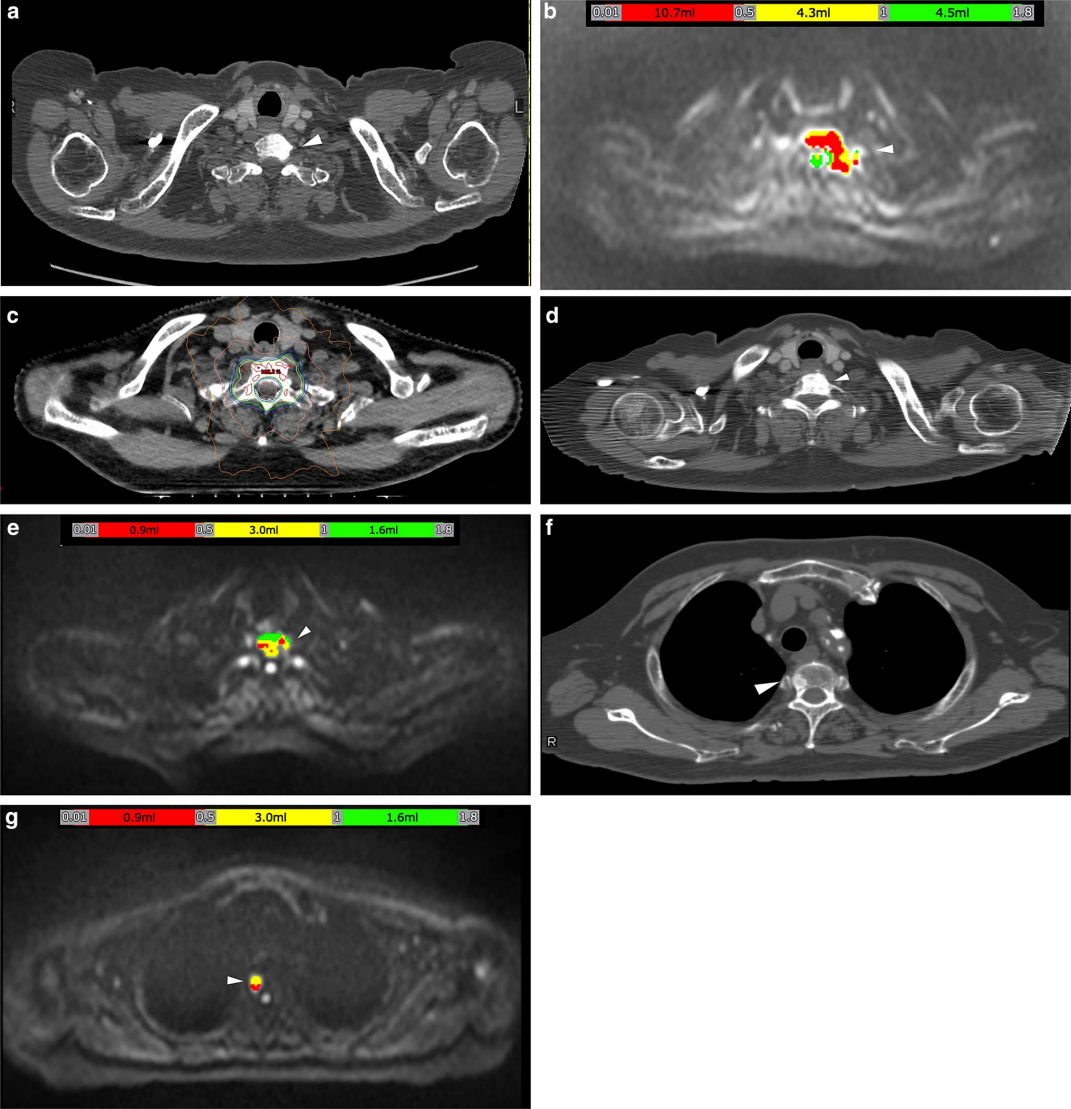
A 70-year-old man with CRPC. Six years ago, local radiotherapy was performed for prostate cancer (T2c N0M0, PSA 32.1 ng/mL, GS 4 + 5). Four years later, the patient’s condition transitioned to CRPC. a On the original axial CT at the level of C7, osteosclerotic change was seen in the left part of C7 body (white arrowhead). At that time, the patient’s PSA level was 4.413 ng/mL. b On the BD score–composed axial image at the level of C7, a colored area was seen (white arrowhead). tDV was 19.5 mL, and the mean ADC value was 0.74 (× 10^–3^ mm^2^/s). c The C7 lesion was regarded a metastatic lesion, intensity-modulated radiation therapy was performed (35 Gy/5fr RT.) with reference to the axial DW image. d–g Approximately 10 months after the image shown in a–c, PSA increased to 6.235 ng/mL. d On the original axial CT at the level of C7, the osteosclerotic change increased in the left part of the C7 body (white arrowhead) from that shown in a. e On the BD score–composed axial image at the level of C7, a colored area was seen (white arrowhead). The colored area decreased from that in b. f On the CT image at the level of Th3, a subtle sclerotic change was newly observed (white arrowhead), but this was a retrospective finding. g On the BD score–composed axial image at the level of Th3, a colored area was newly observed in the right part of the body (white arrowhead). In this case, tDV decreased to 5.5 mL, and consequently, mean ADC increased to 0.83 (× 10^–3^ mm^2^/s); however, Th3 was diagnosed as a new metastatic lesion
